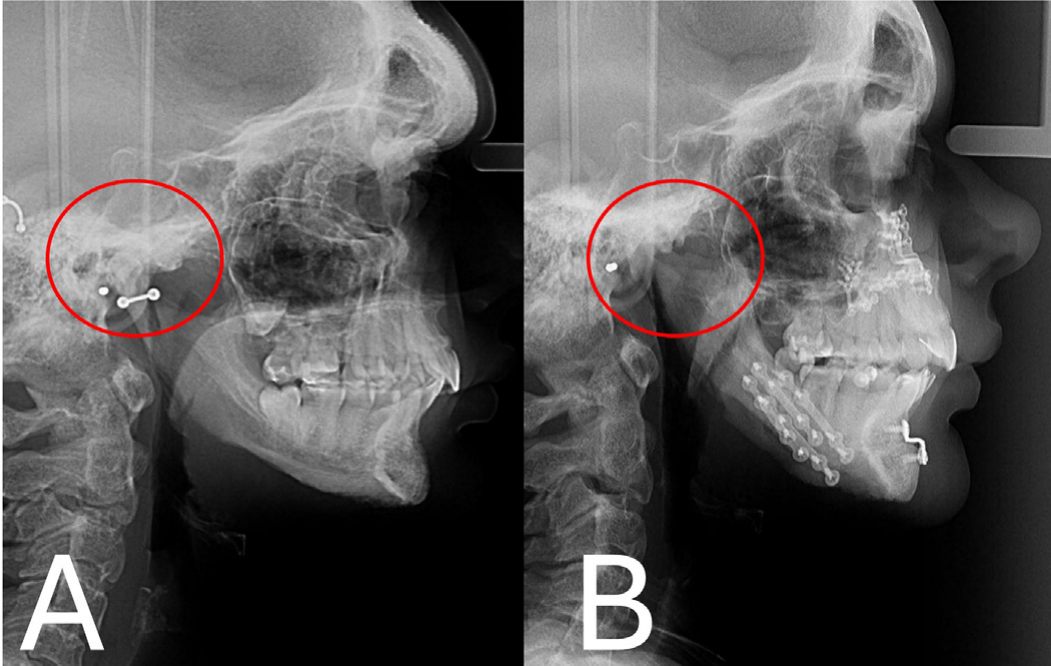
Lateral radiograph (A) before orthognathic surgery and (B) 2 years after surgery showed the occlusal relapse and retrognathia.
